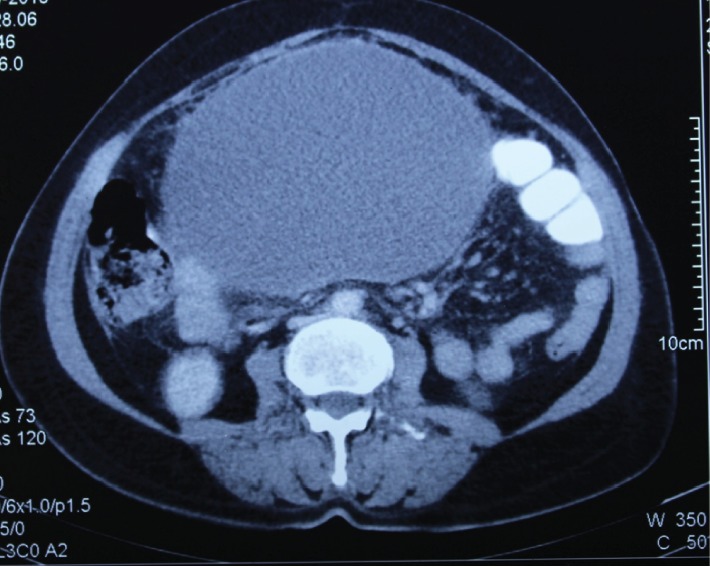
CT transverse plane of the abdomen showing a huge cystic lesion of 19 × 14 × 12 cm.
